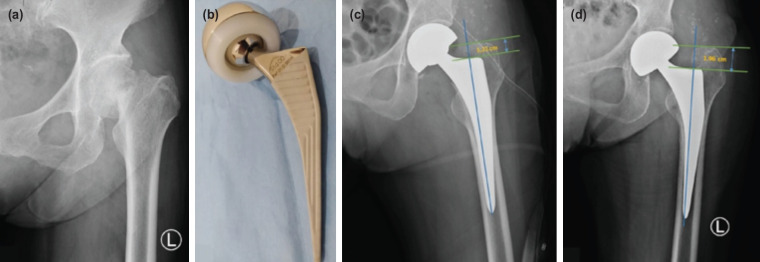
(a) Example of measured femoral stem subsidence in a female, 79 years old. Pre-operative radiograph. (b) Implants that was used in this study. (c) Post-operative radiograph. (d) Radiograph taken during follow-up (three months).
